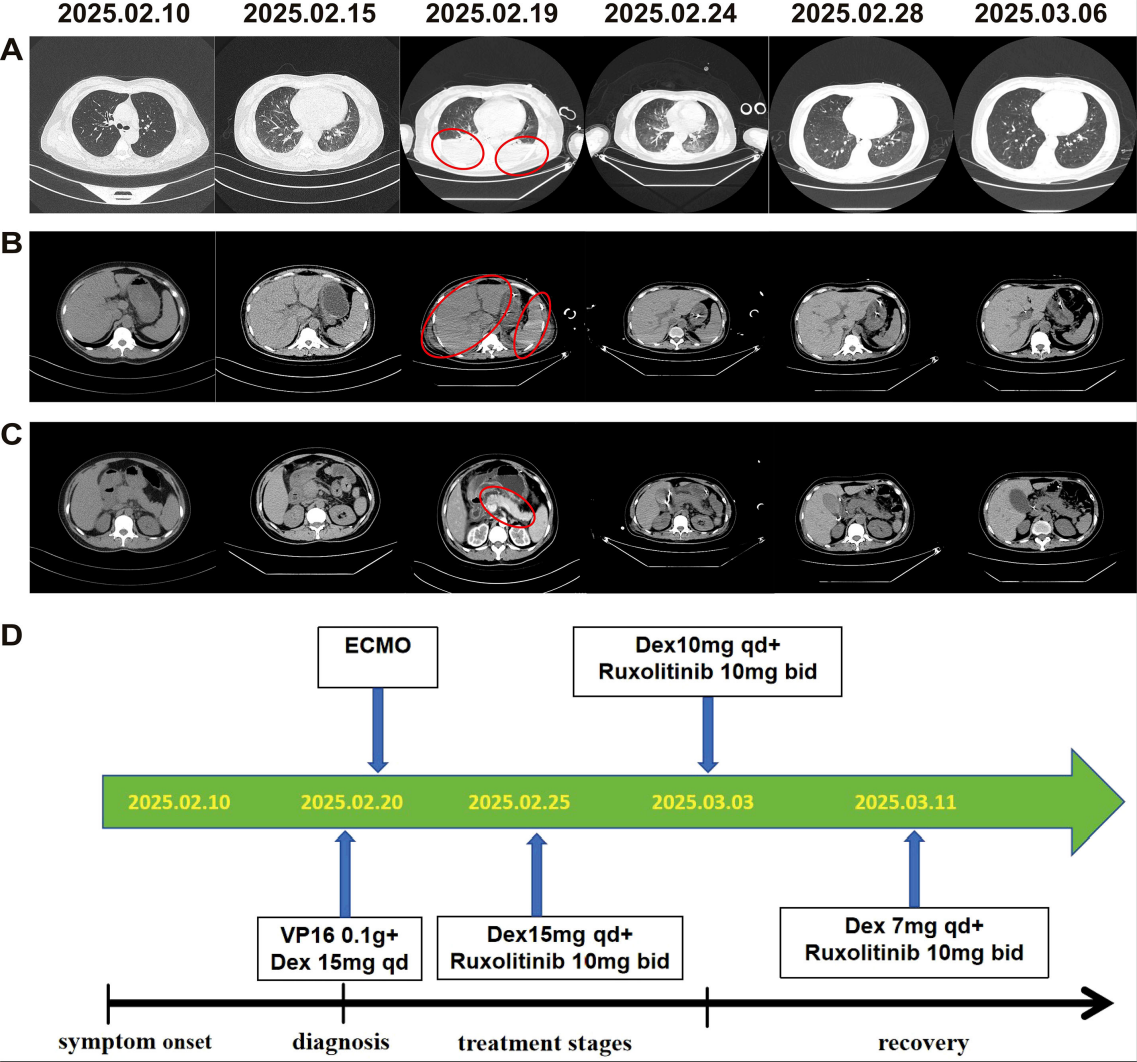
Time course of treatment and computed tomography findings. (A) The lung (new infectious lesions and massive pleural effusion, red circle); (B) the liver and spleen (enlargement, red circle); (C) the pancreas (acute pancreatitis, red circle); (D) the timeline of the patient’s treatment. Dex, dexamethasone; ECMO, extracorporeal membrane oxygenation.
